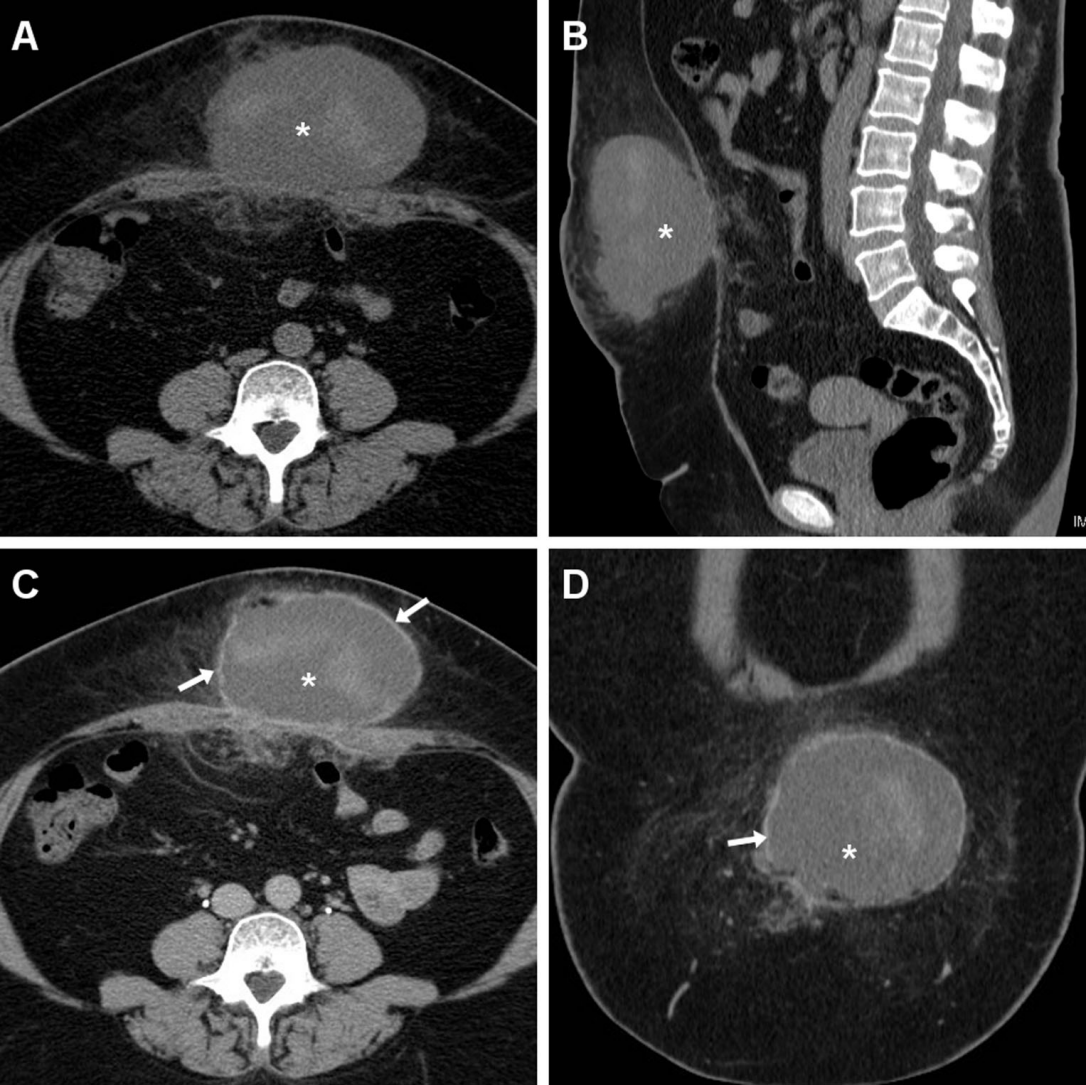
Post-surgical haematoma developed within three days after VHR in a 44-year-old female, with physical finding of postoperative swelling at the anterior abdominal wall. Unenhanced axial (a) and sagittal (b) images showed a demarcated midline collection with heterogeneous attenuation (*) abutting the rectus muscles externally and occupying the subcutaneous fat. Axial (c) and coronal (d) post-contrast CT images showed minimal peripheral enhancement (arrows), without signs of active bleeding
